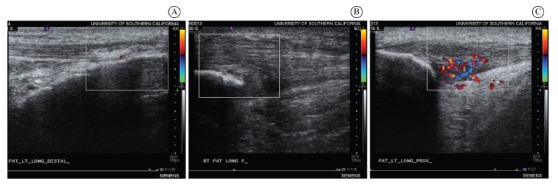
Ultrasound images of the patellar tendon: (A) distal patellar tendon
